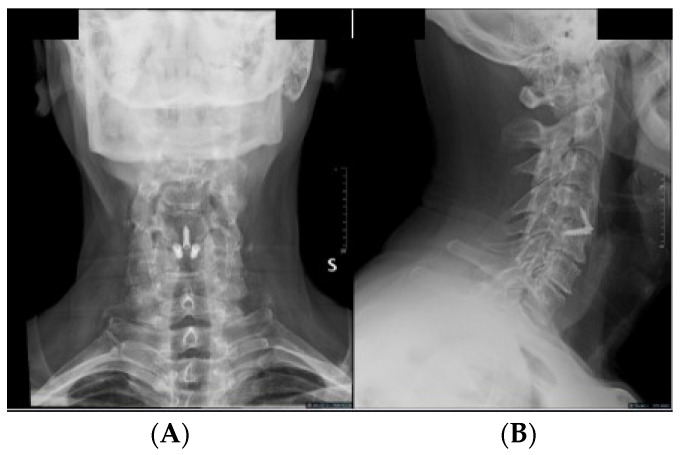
Postoperative (A) AP and (B) lateral X-ray displaying a C4-5 ACDF using an interfixated zero-profile PEEK cage.
